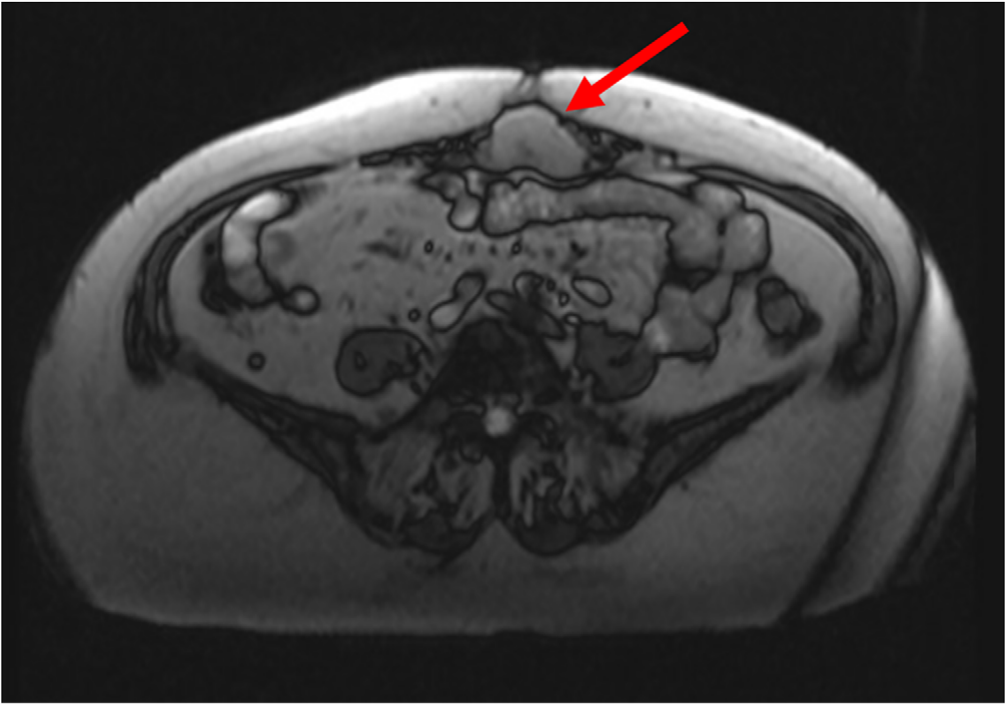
T2‐weighted magnetic resonance imaging (MRI) abdomen images. Red arrow indicates abdominal wall neuroendocrine tumor (NET)
